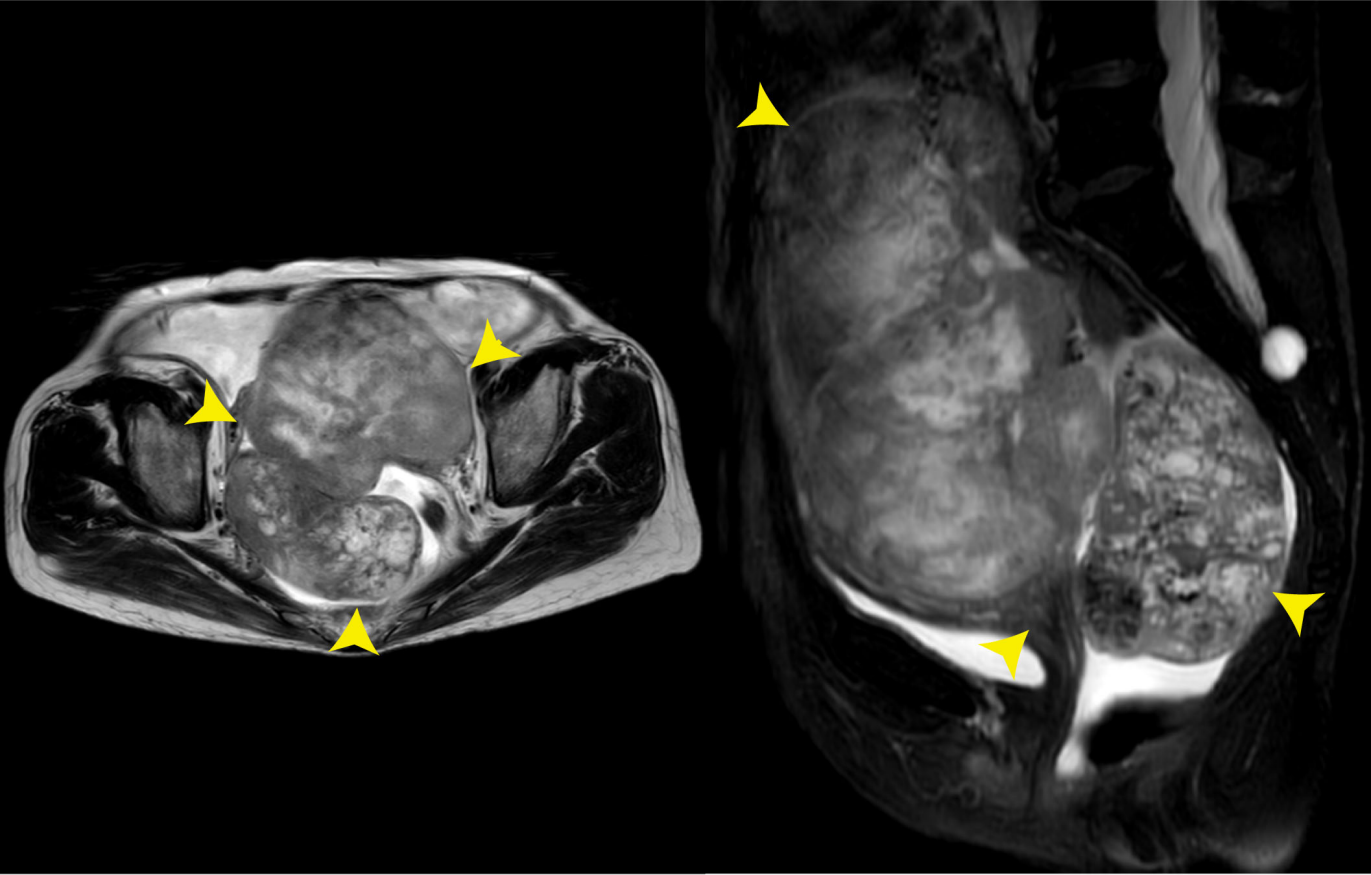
Pelvic MRI on 18 August 2020. The images showed an expansive heterogeneous malignant tumor in the lower abdominal and pelvic cavity (yellow arrows).
